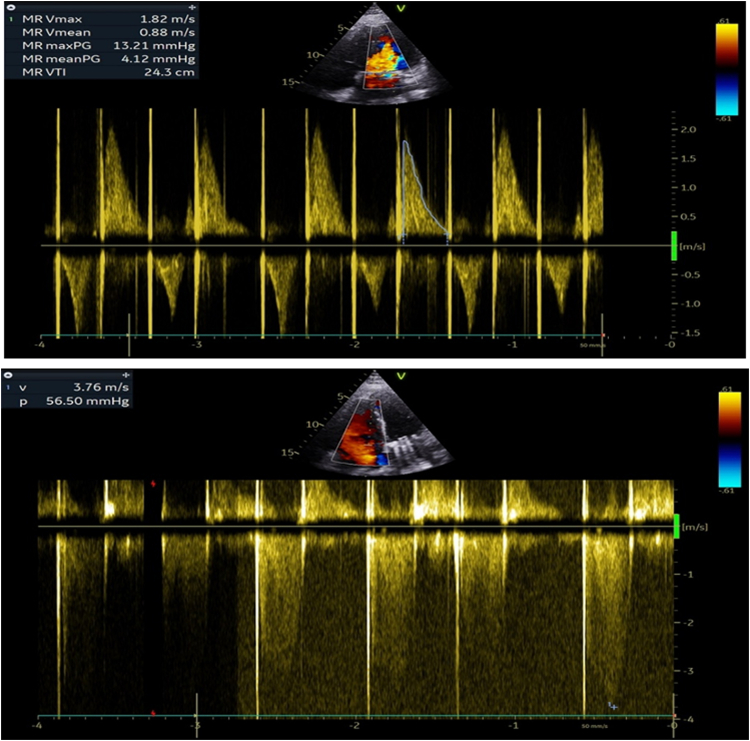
Postoperative Echocardiographic Assessment
